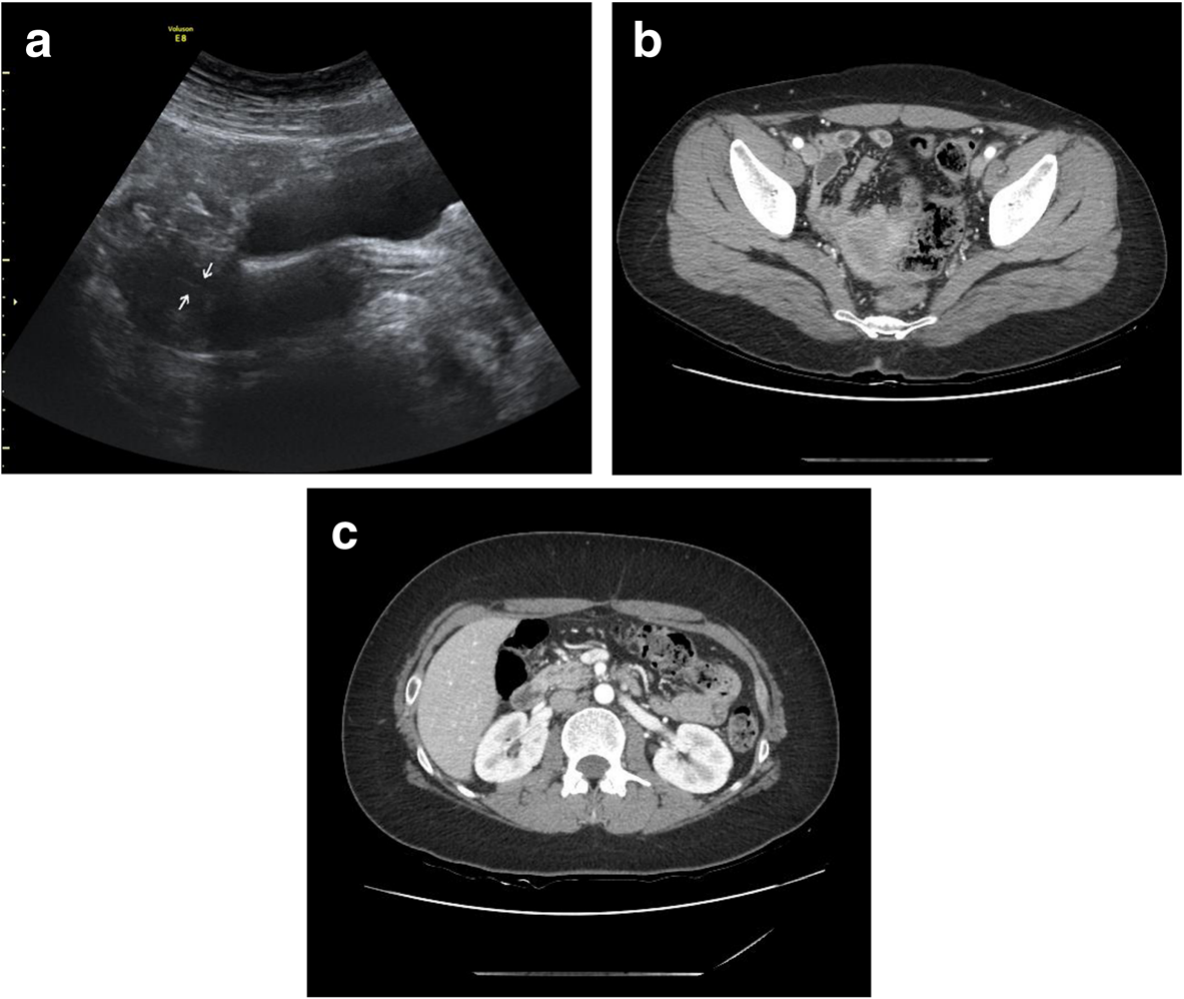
Ultrasonography image (a) and abdomen computed tomography axial image with contrast enhancement (b) shows small uterus and both ovaries are not visible. There was no abnormalities of urogenital system (c)
